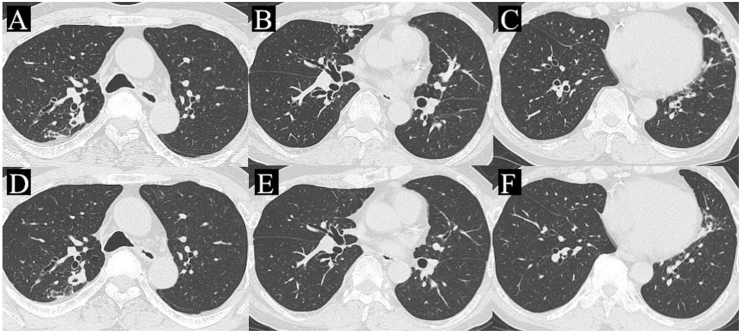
Chest CT, acquired three months after the discontinuation of treatment, demonstrates the increased nodular shadow in the upper lobe (A) and the newly appeared, bilateral, centrilobular nodules in the lingular segments (B, C). After four months of clarithromycin and rifampicin administration, these improved (D–F). CT: computed tomography.
